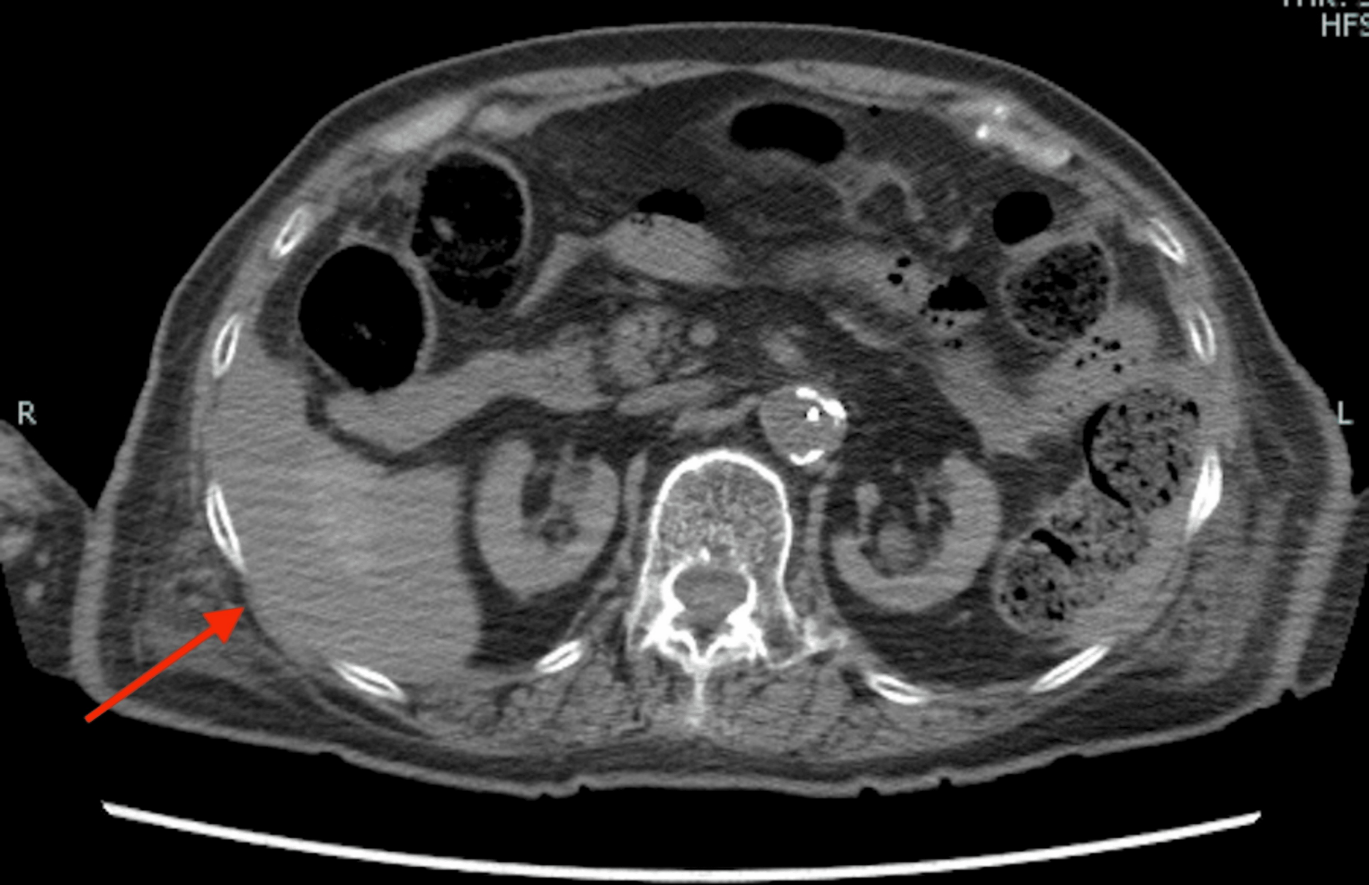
CT image (abdomen) CT scan showing hemoperitoneum with evidence of hepatic lacerations.
